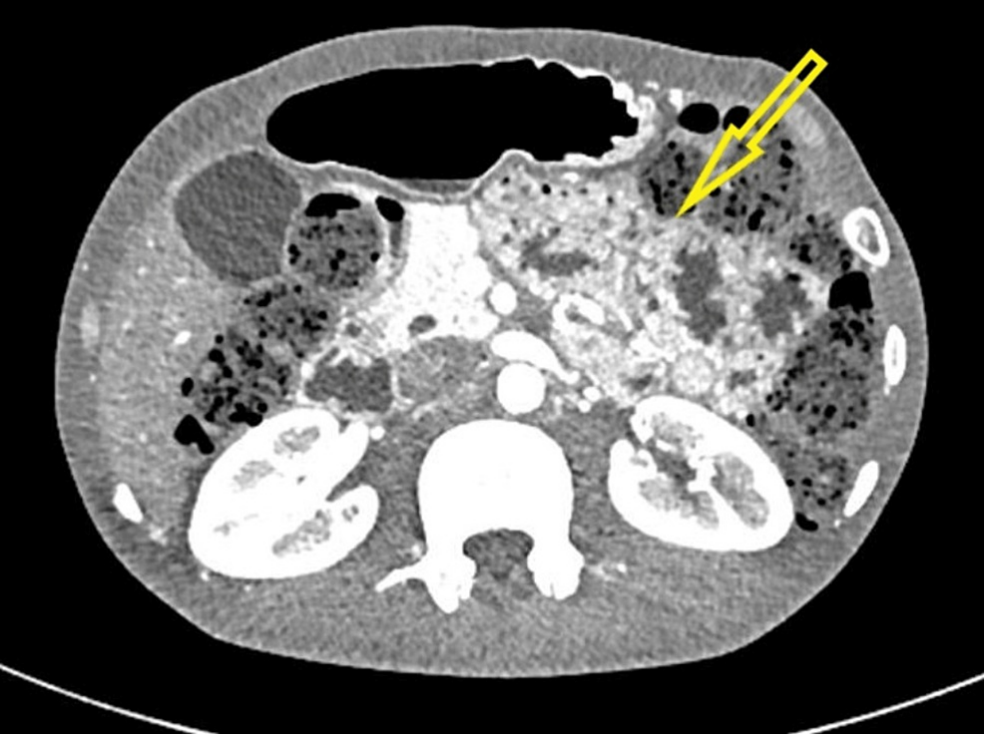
CECT of the abdomen showing small bowel loops occupying the region of the pancreatic body and tail (hollow arrow) CECT: contrast-enhanced computed tomography
